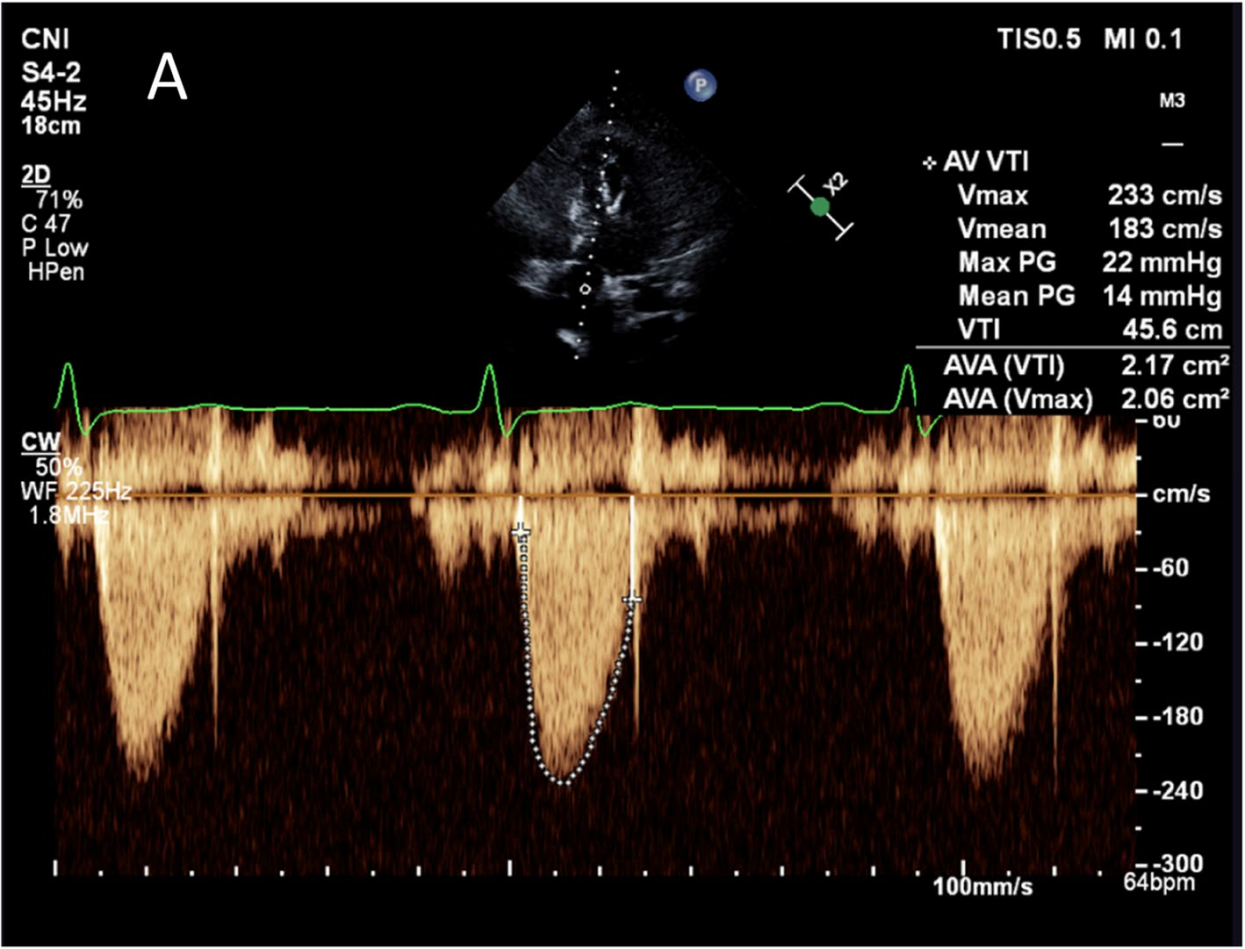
Postoperative transthoracic echocardiography. Normal aortic bioprosthetic valve hemodynamic
